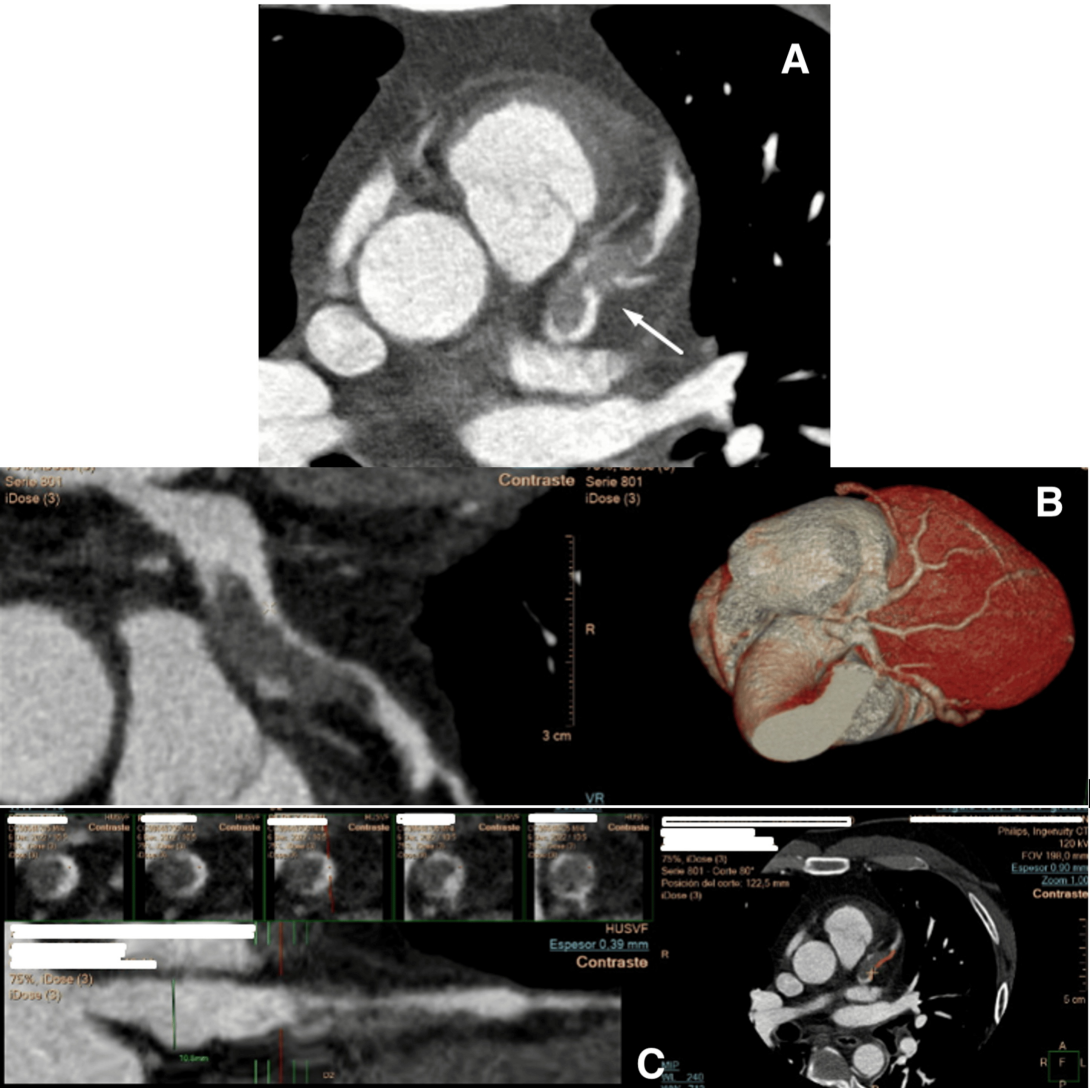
Coronary CT angiography showing a left main coronary artery aneurysm extending into the left anterior descending artery, with an acute intraluminal thrombus causing 80% luminal stenosis. (A) Axial CT angiographic image demonstrating aneurysmal dilatation of the left main coronary artery (arrow). (B) Curved multiplanar reconstruction of the left coronary artery combined with a 3D volume-rendered reconstruction, clearly depicting the aneurysm morphology and its extension into the left anterior descending artery. (C) Sequential cross-sectional views along the coronary lumen reveal the presence of intraluminal thrombus, responsible for approximately 80% luminal stenosis.
